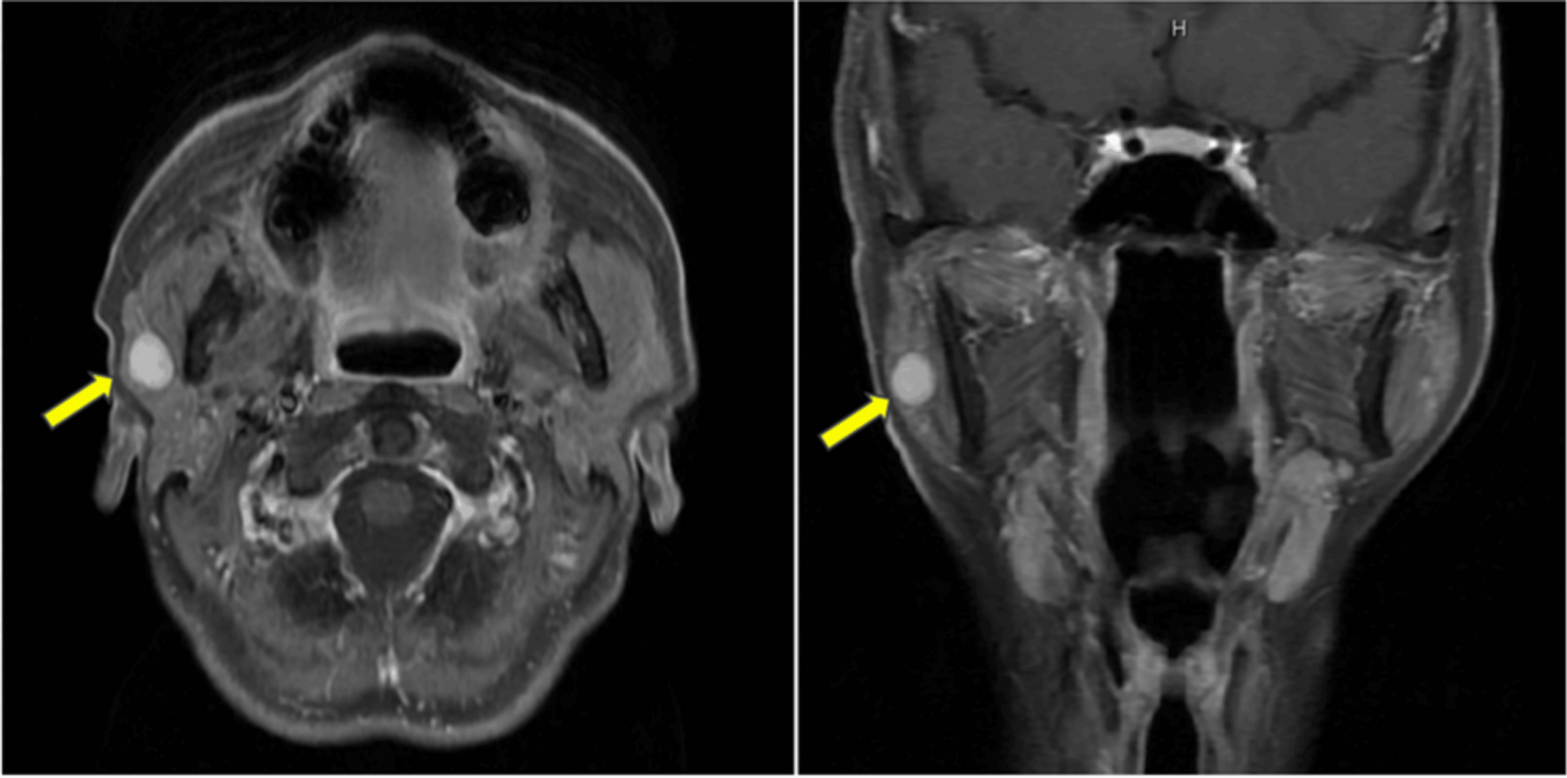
Preoperative T1-weighted gadolinium-enhanced MRI image The yellow arrow indicates the tumor. A mass with a well-defined margin measuring 10 × 14 × 12 mm and showing homogeneous contrast enhancement is noted in the right preauricular region.
